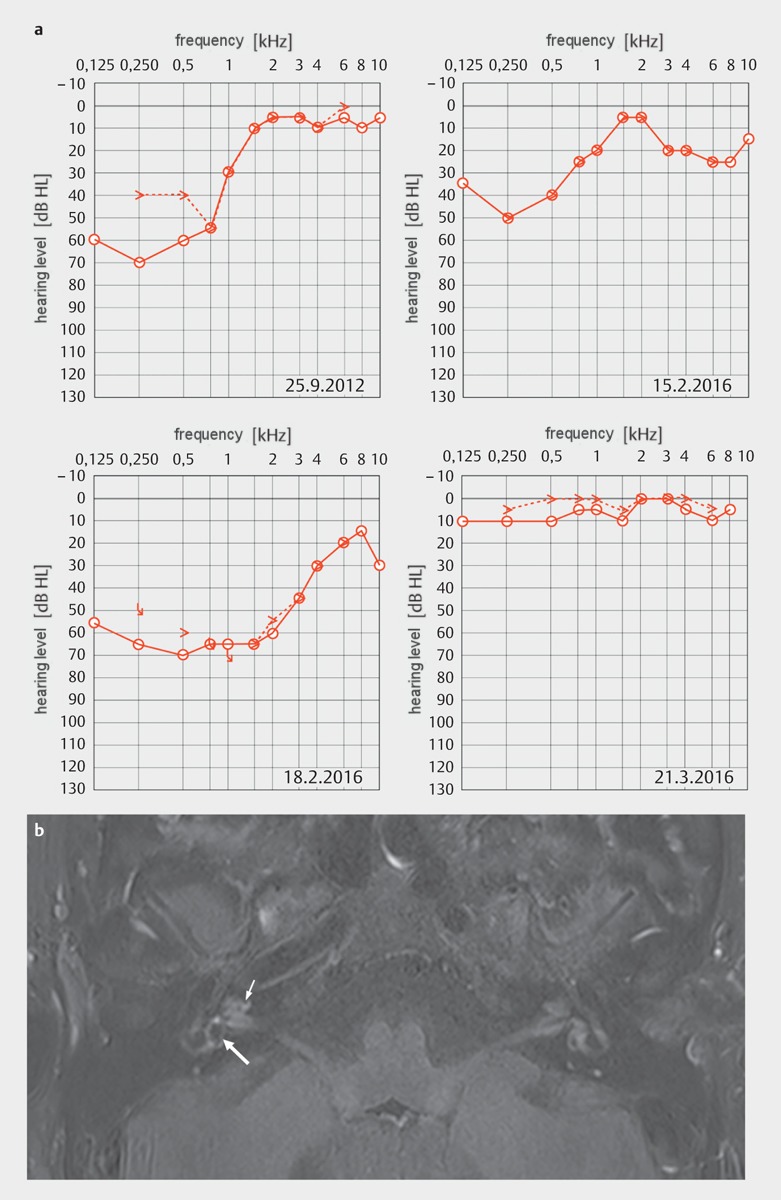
Hydropic ear disease, right-sided: a: The patient suffered from “recurrent sudden hearing losses” in the lower frequency range on the right side, which recovered during systemic high-dose prednisolone therapy (“fluctuating hearing loss”). Considering the imaging (b), the disease is classified as “primary hydropic ear disease of cochlear type” and when vertigo appears as “primary hydropic ear disease of the cochlea-vestibular type” or Menière’s disease [46]. b: MRI reveals an enlarged endolymphatic space in the cochlea (small arrow) and in particular in the vestibulum (large arrow) of the right side, indicating a moderate endolymphatic hydrops (3D IR sequence, 6 hours after intravenous application of contrast medium); CM: contrast agent; w: weighted. (b Department of Radiology, University Medicine Halle, courtesy of Prof. Dr. S. Kösling).
